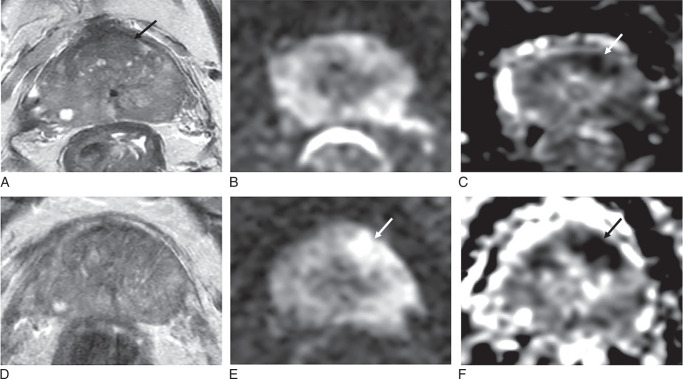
Differential scoring prone versus supine imaging. Seventy-one-year-old patient, PSA 7.31 ng/mL. A–C, Supine imaging shows 13 mm area of homogeneous low T2 signal in the left mid-anterior TZ with partial encapsulation, PI-RADS 2 (arrow, A), DWI, PI-QUAL 2/4 (B, C), shows marked low signal on ADC (arrow, C), but no significant change on b-1400 DWI (B); overall PI-RADS 3. DCE imaging in the TZ was negative (not shown). D–F, Prone T2 is nondiagnostic, PI-QUAL 0/4 (D), DWI shows 28 mm area of marked restricted diffusion (arrows) on b-1400 DWI (E) and ADC map (F), PI-RADS score 5. Overall, PI-RADS score 3 (2+1) using supine T2 and prone DWI, PI-RADS score 5 using prone imaging alone. Targeted biopsy shows Gleason 3+3=6 in 3/3 cores with 13 mm max tumor length.
